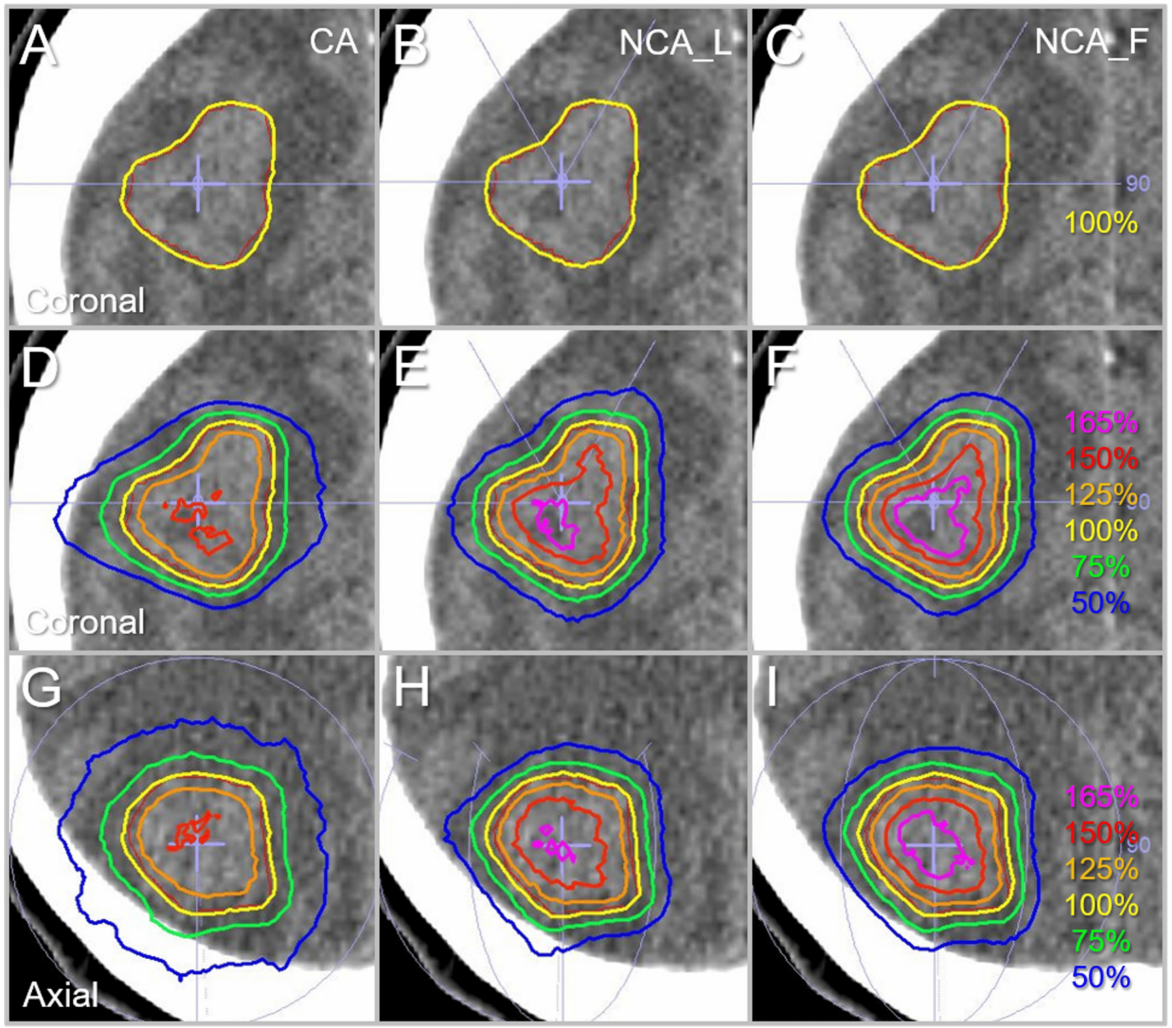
Comparison of the dose distributions for the GTV of 9.54 cc. The images show head CT images of a patient with a single BM (A-I), onto which the GTV contoured in red, arc arrangements, and representative isodoses are superimposed; coronal views with the most irregular GTV shape (A-F); and axial views (G-I). The isodose lines are shown as relative values with the GTV *D*_V-0.01 cc_ as 100% (yellow). GTV: gross tumor volume; CA: coplanar arcs; NCA_L: non-coplanar arcs with limited rotations; NCA_F: non-coplanar arcs with full rotations; CT: computed tomography; BM: brain metastasis; *D*_V-0.01 cc_: a minimum dose covering a target volume minus 0.01 cc.
